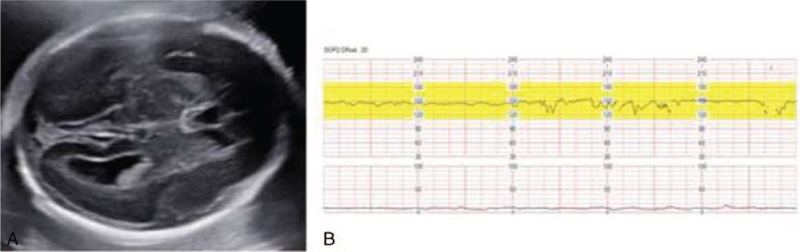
Data from examinations the day before fetal brain hemorrhage. (A) Ultrasonography of fetus conducted the day before fetal brain hemorrhage was diagnosed. No signs of fetal brain abnormalities including brain hemorrhage were evident. (B) Nonstress test (NST) showed moderate to good variability. The mother did not complain about decreased fetal movement.
